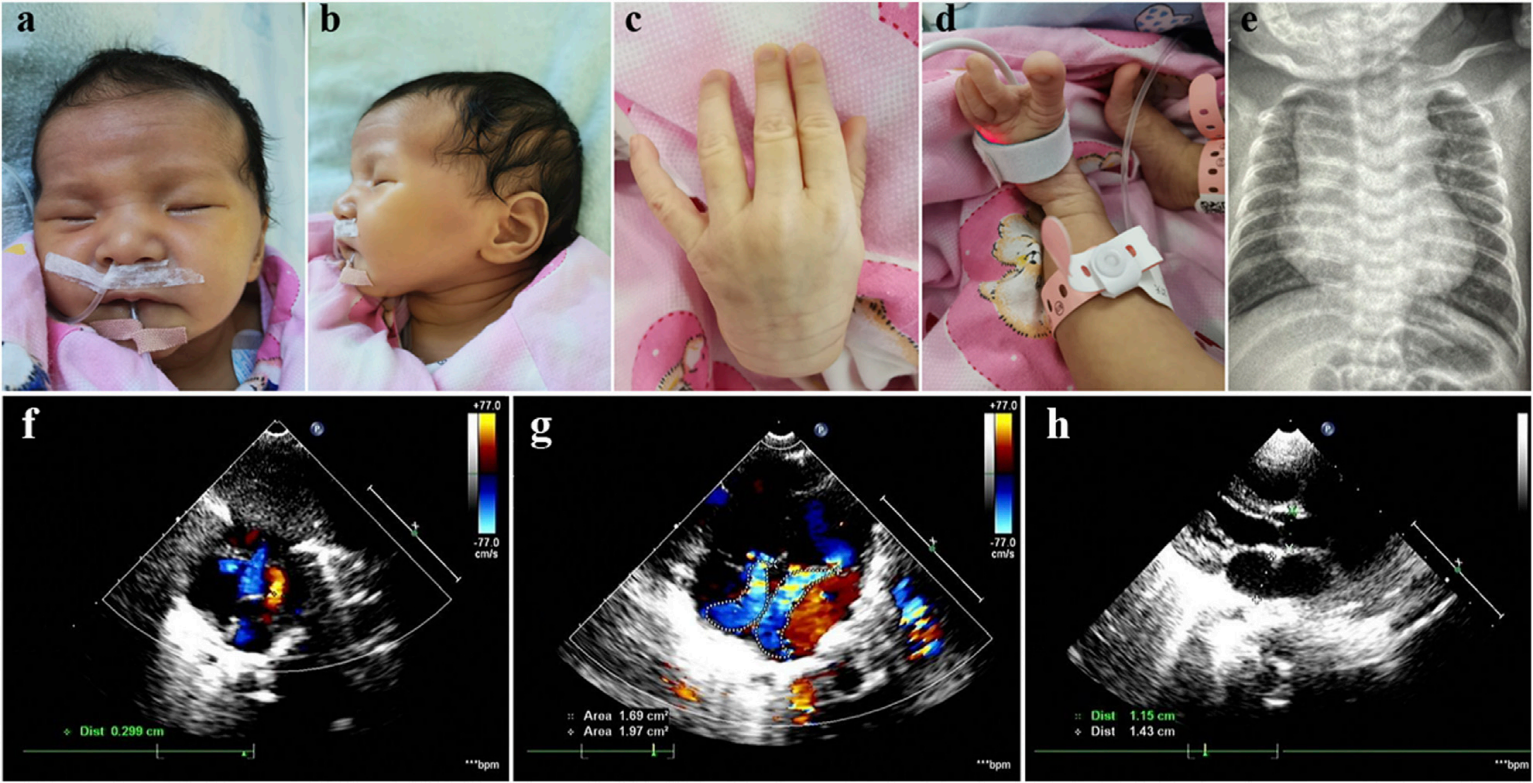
Photographs of our patient with MFS. Note bilateral temporal skull flattening, enophthalmos, retrognathia, arachnodactyly, pes planus, long, narrow feet, hammer toes and skin striae. (A–D) X-ray showed significant enlargement of the heart shadow with a cardiothoracic ratio of 0.67 (normal value < 0.6) (E). Echocardiography showed atrial septal defect, mitral regurgitation, tricuspid regurgitation and dilatation of the aortic root, 15 mm with Z-score of 4.48 (F–H).
